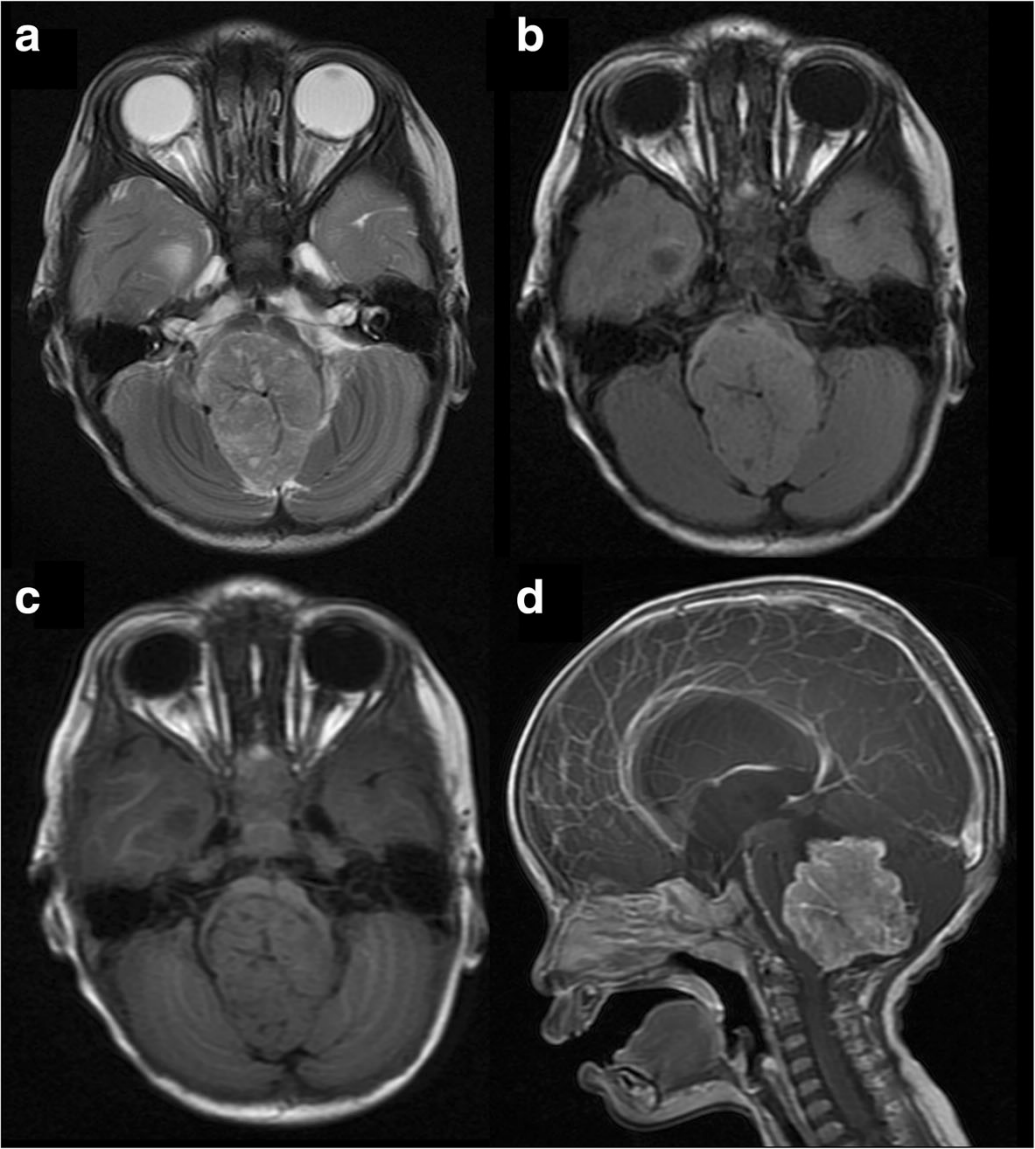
aCPP (grade II). A 2-year-old girl with a lobulated lesion in the fourth ventricle. The tumour is slightly hyperintense on T2WI (a) and FLAIR sequence (b) and isointense on T1WI (c), with a more heterogeneous internal structure. Sagittal post-contrast imaging shows that the tumour is strongly enhanced (d), and serious hydrocephalus is visible in the supratentorial ventricles
